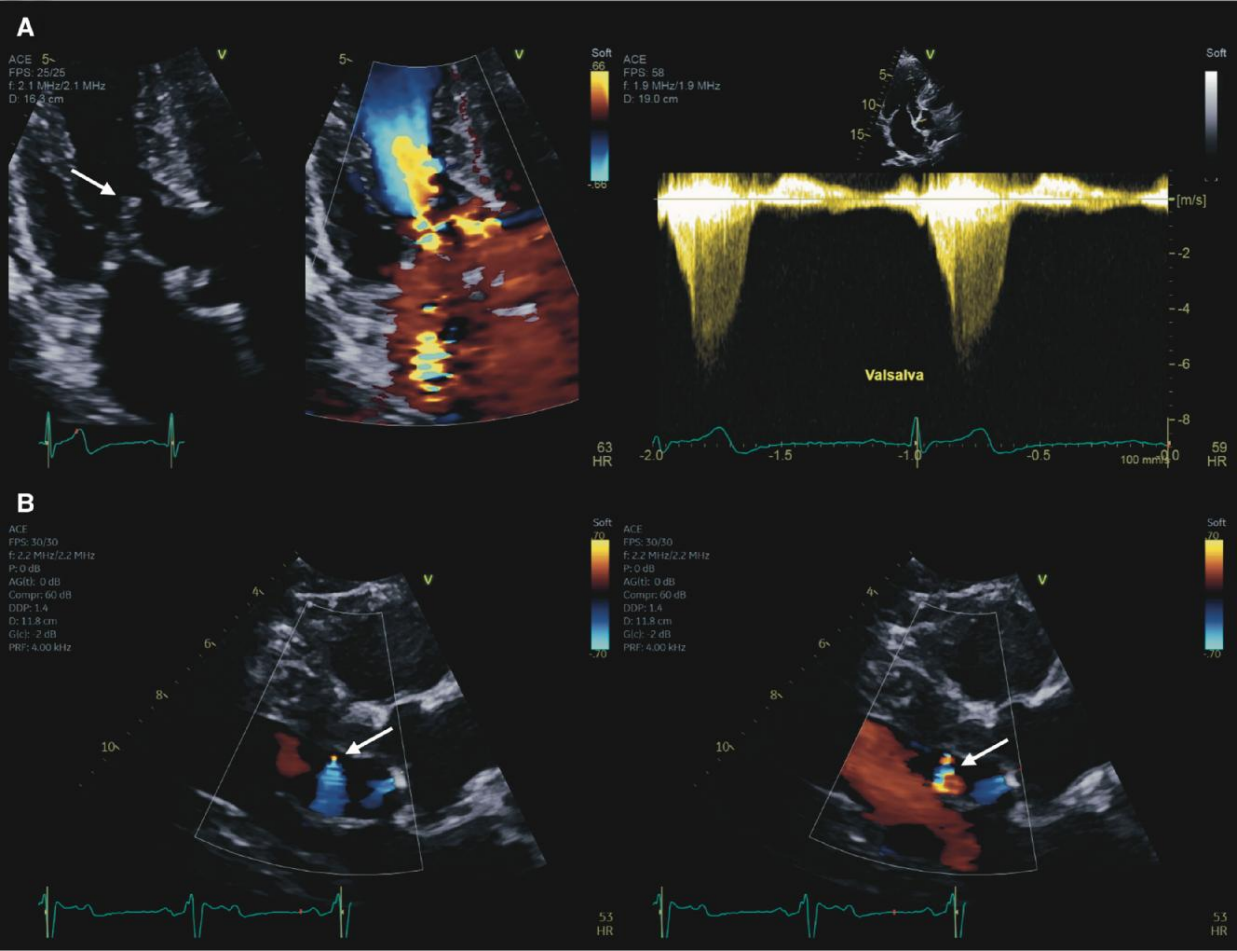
(*A*) Pre-surgical echocardiography demonstrating septal hypertrophy and dynamic left ventricular outflow obstruction (right upper panel) due to systolic anterior motion of the mitral valve (left upper panel, arrow). (*B*) Post-surgical colour Doppler demonstrating flow from the ventricular septum to the left ventricular cavity (arrows). Additional echocardiography imaging is available in the Supplementary material online.
